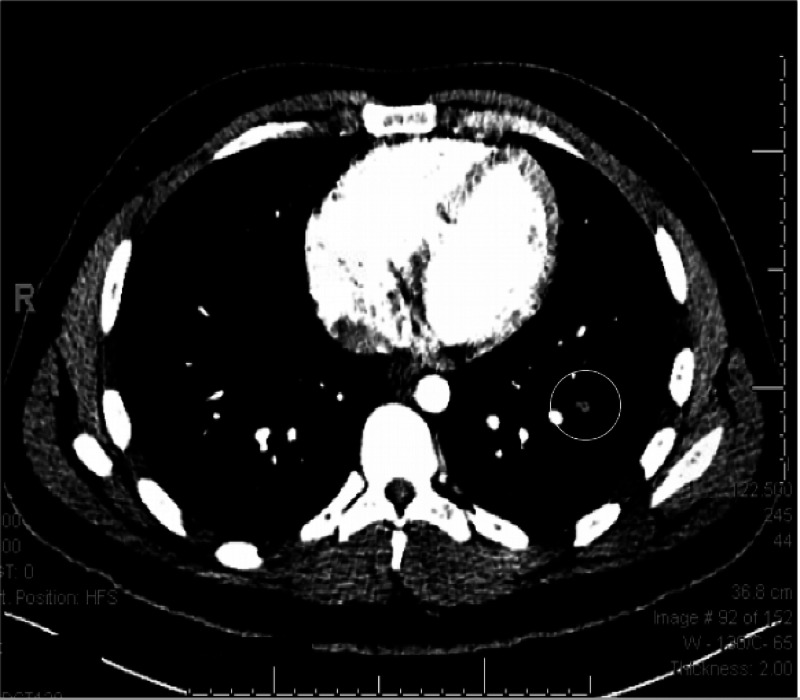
CT scan of the chest with contrast (axial view) showing a filling defect in the left lower lobe segmental and subsegmental pulmonary arteries consistent with pulmonary embolism.
